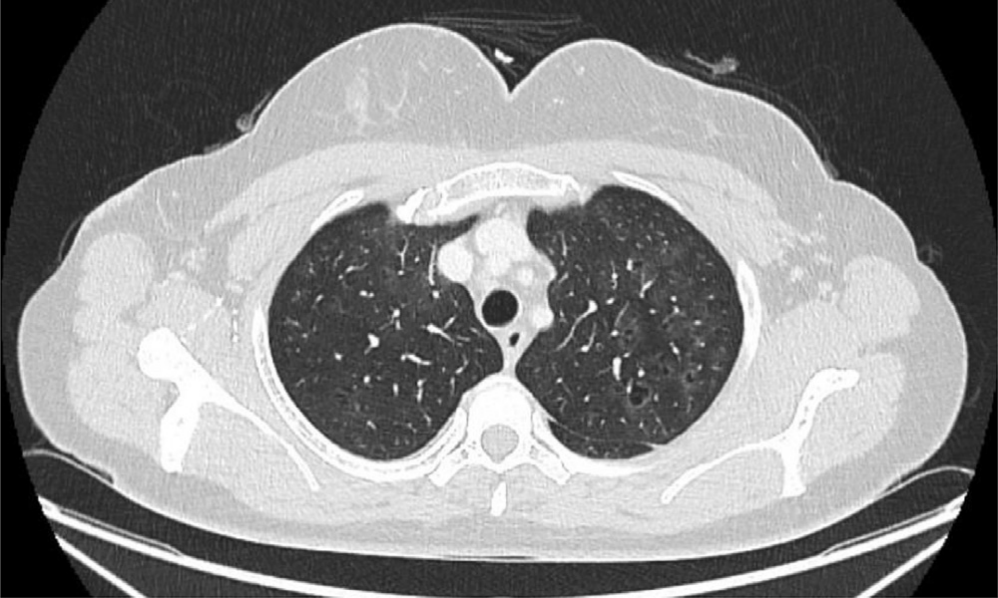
Apical slice of CT thorax demonstrating subtle peribronchovascular groundglass opacification predominantly within the upper lobes and mild upper lobe predominant centrilobular emphysema.
